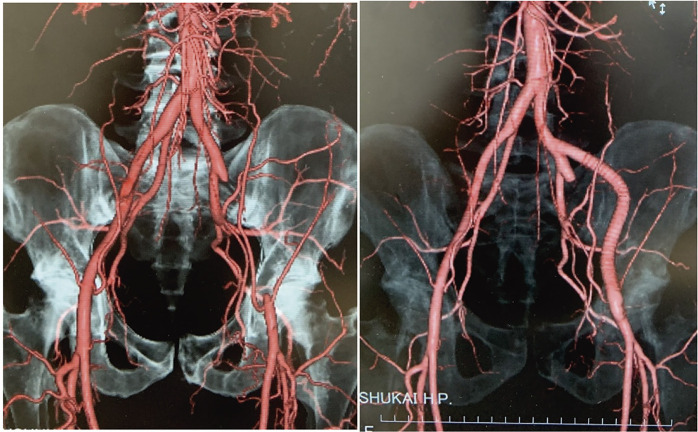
### P2-3 Impact of Metabolic Activity on Abdominal Aortic Aneurysm Growth Evaluated by ^18^F-FDG PET–CT
